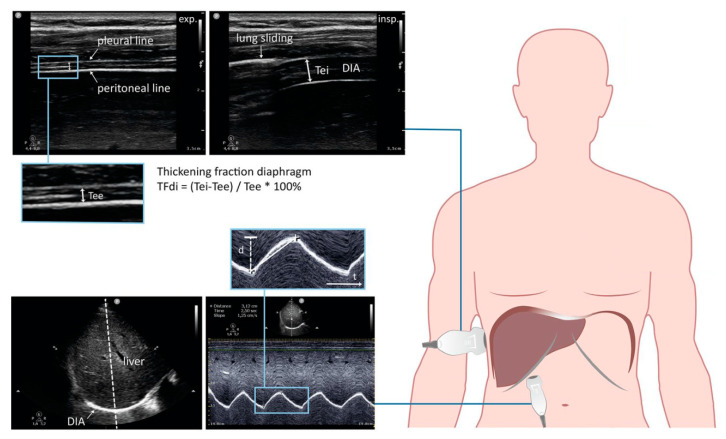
Clinical application of diaphragm muscle ultrasound (Figure modified according to: Tuinman et al. [14], Figure 1; p. 596).
